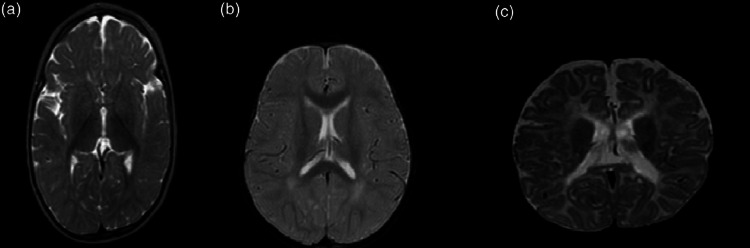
The magnetic resonance imaging findings of the patients. (a) Axial T2-weighted image has mild atrophy in the temporal area and delayed myelination. (b) Axial T2-weighted image has the delayed myelination in the periventricular areas. (c) Axial T2-weighted image shows mild cerebral atrophy and thinning of the corpus callosum.
